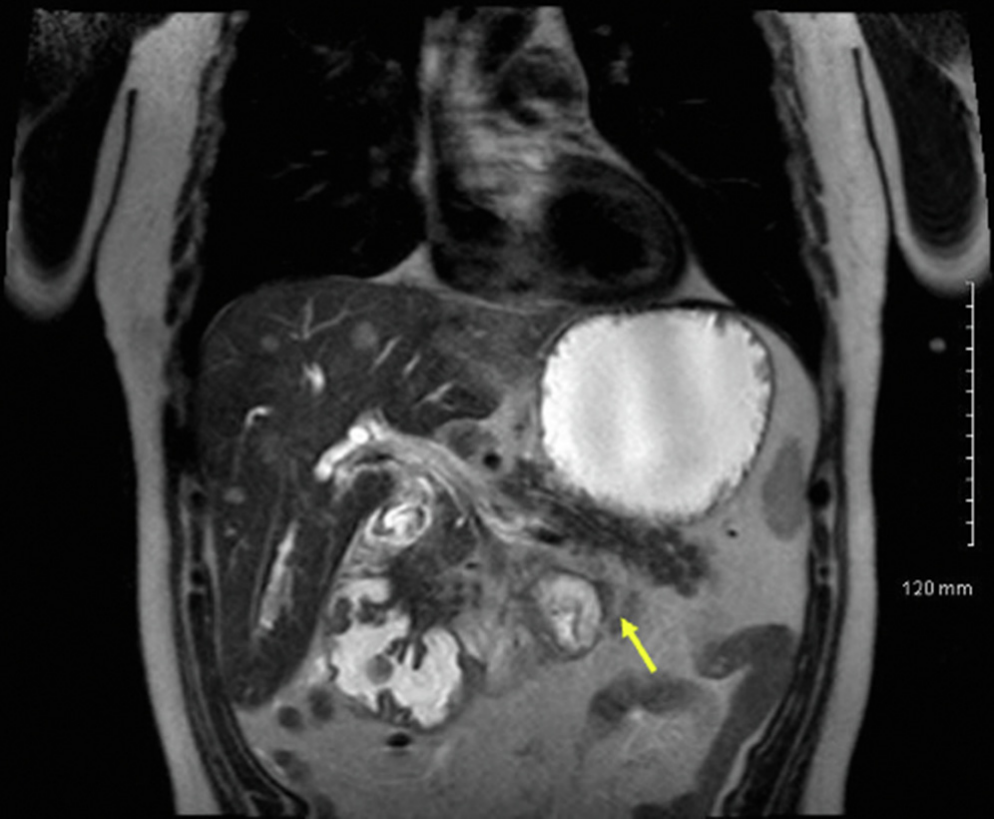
MRI scan of abdomen showing circumferential obstructing mass in the proximal jejunum (*arrow*).
